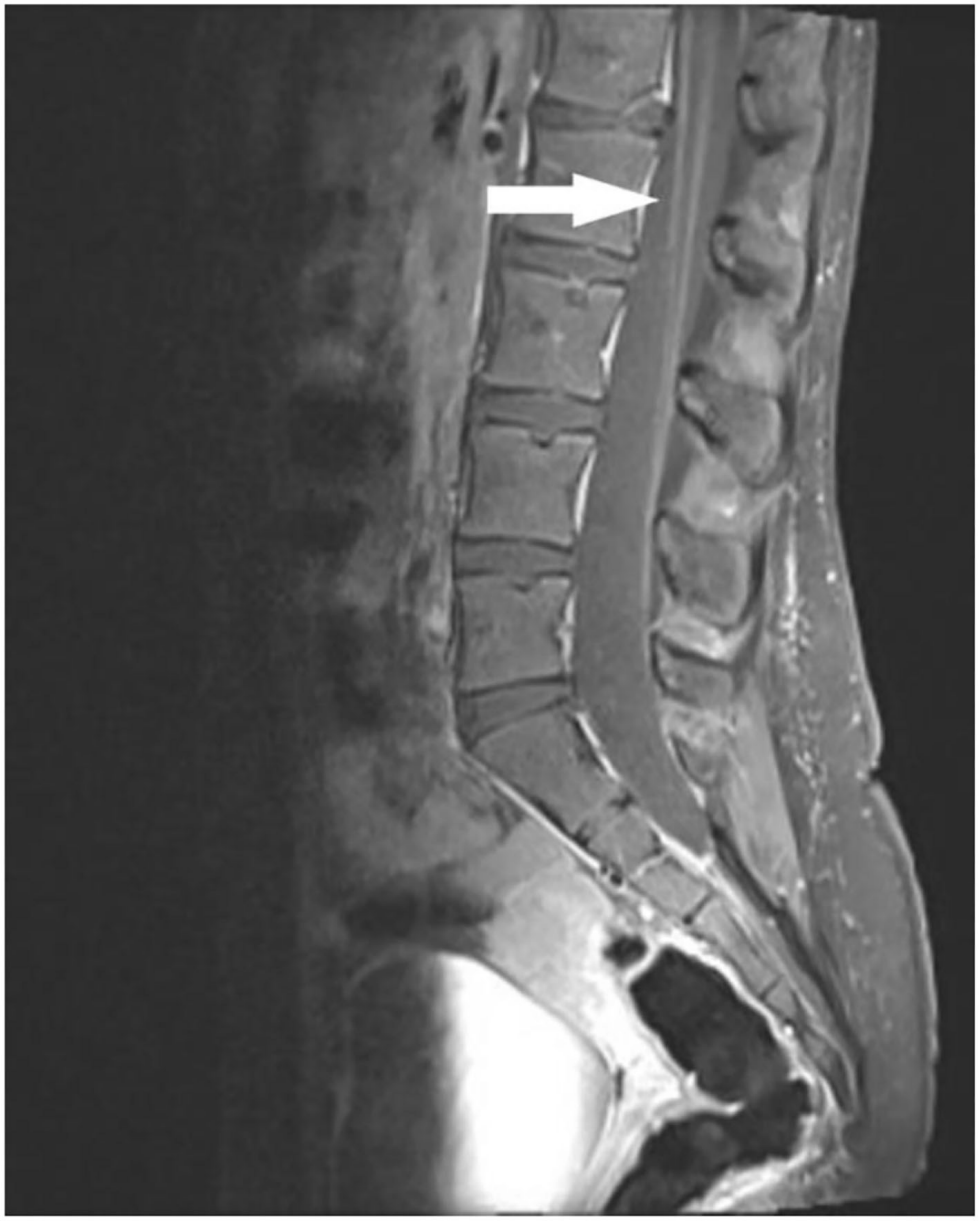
Sagittal post-contrast T1 weighted MRI image of lumbo-sacral spine demonstrating cauda equina root enhancement (arrow).
